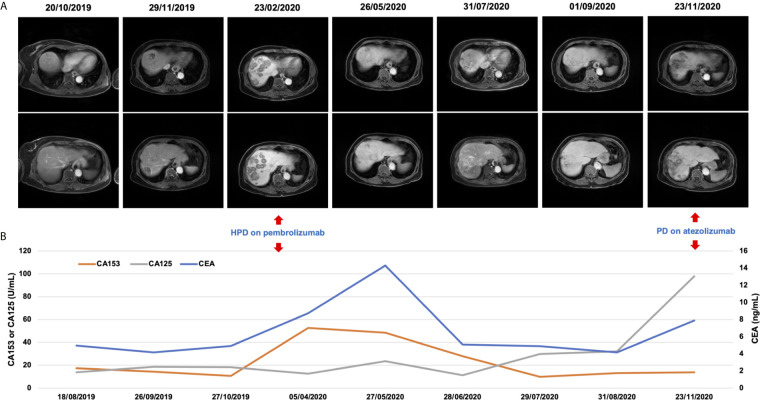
Radiological evaluation and altered levels of circulating tumor markers before and after HPD. (A) After receiving immunotherapy with pembrolizumab, this patient developed HPD. An MRI scan showing more extensive and large metastatic lesions in the liver, which responded well to rechallenge with atezolizumab in May 2020, and necrotic areas. (B) Dynamics of the levels of circulating tumor markers (CEA, CA125, and CA153) before and after HPD.
